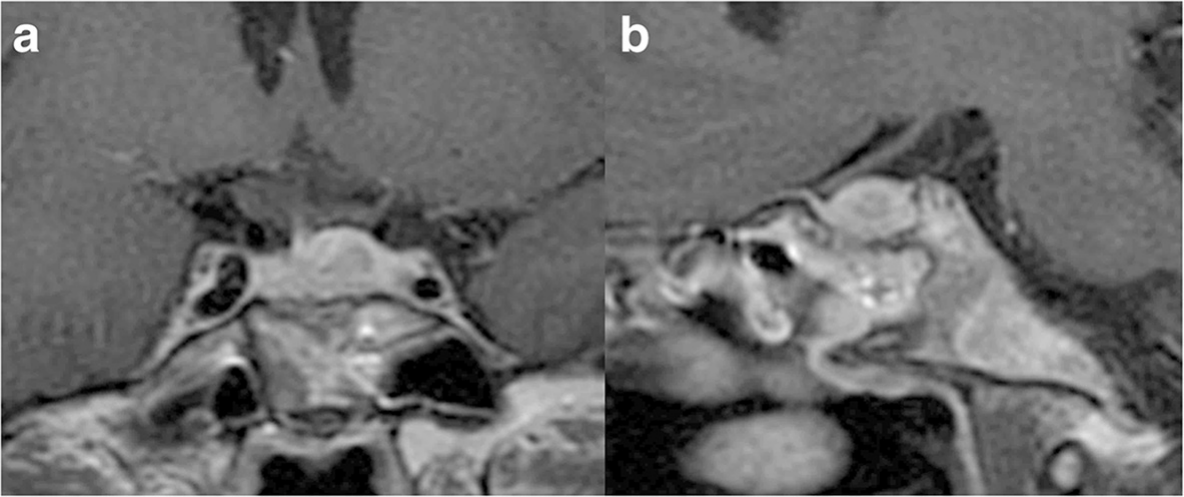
Preoperative MR images of Case 3. Preoperative coronal (a) and sagittal (b) T1-weighted MR images with gadolinium revealing a sellar lesion enhanced heterogeneously with less enhancement in the central portion of the lesion.
